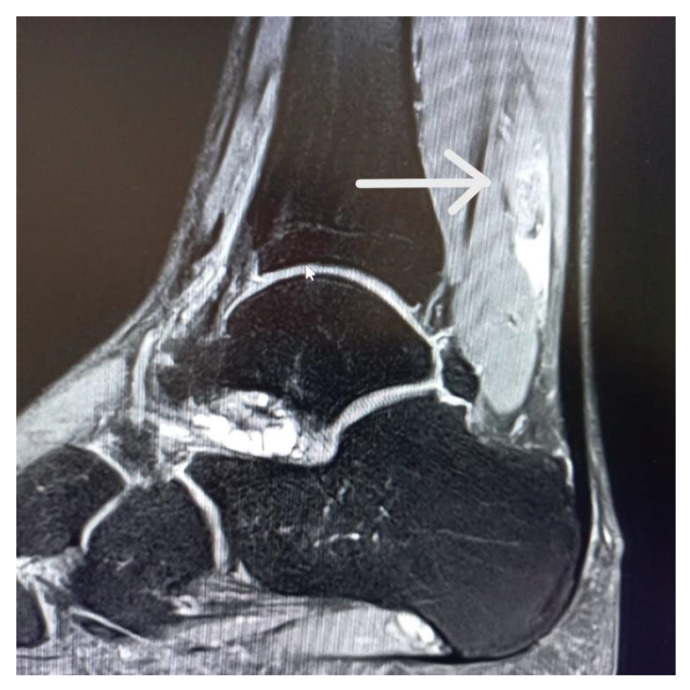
MRI scan 2 weeks after injury.
